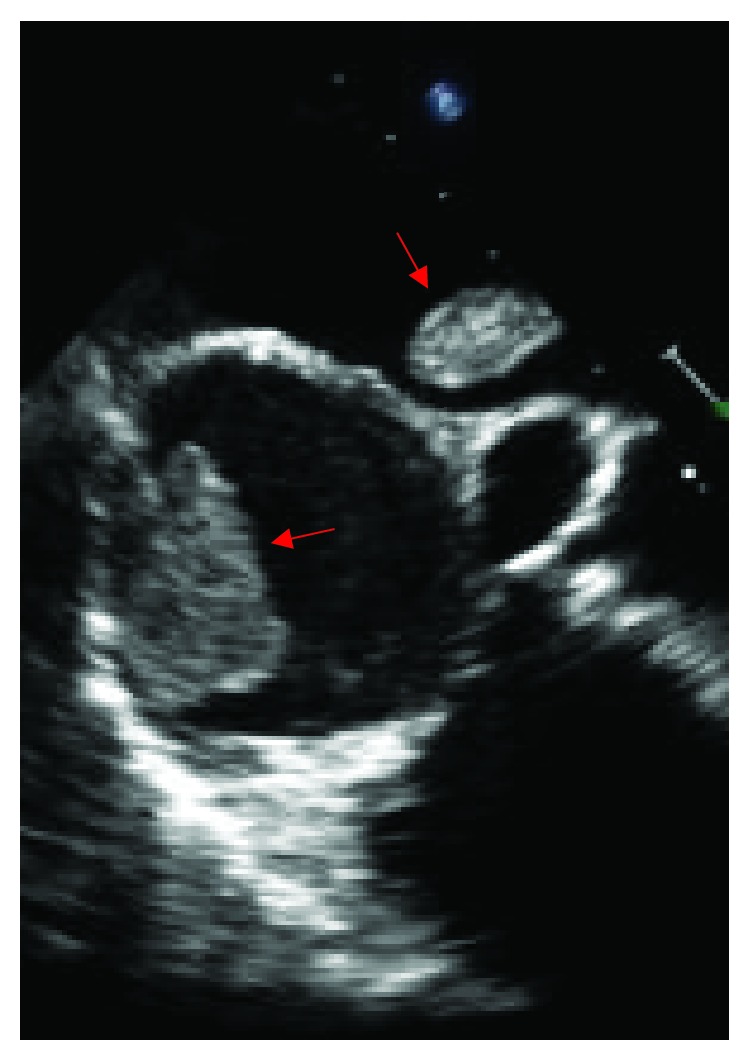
Zoomed-in transesophageal echocardiogram 4-chamber view of left and right atrial thrombi.
